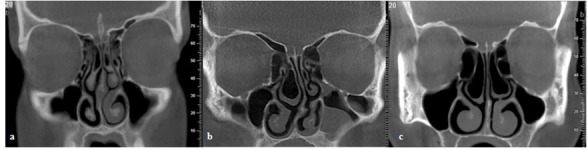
a: Coronal cone bean CT image showing a case with bilateral concha bullosa, variations in superior attachment of both uncinate process (attachment to nasal roof), left uncinate process pneumatization, left paradoxical middle turbinate, Haller cell in the right side and nasal septum deviation  b: Coronal cone bean CT image showing a large right concha bullosa which caused significant nasal septal deviation to the right side.  c: Coronal cone bean CT image showing relatively symmetrical large bilateral concha bullosa and almost straight nasal septum.
